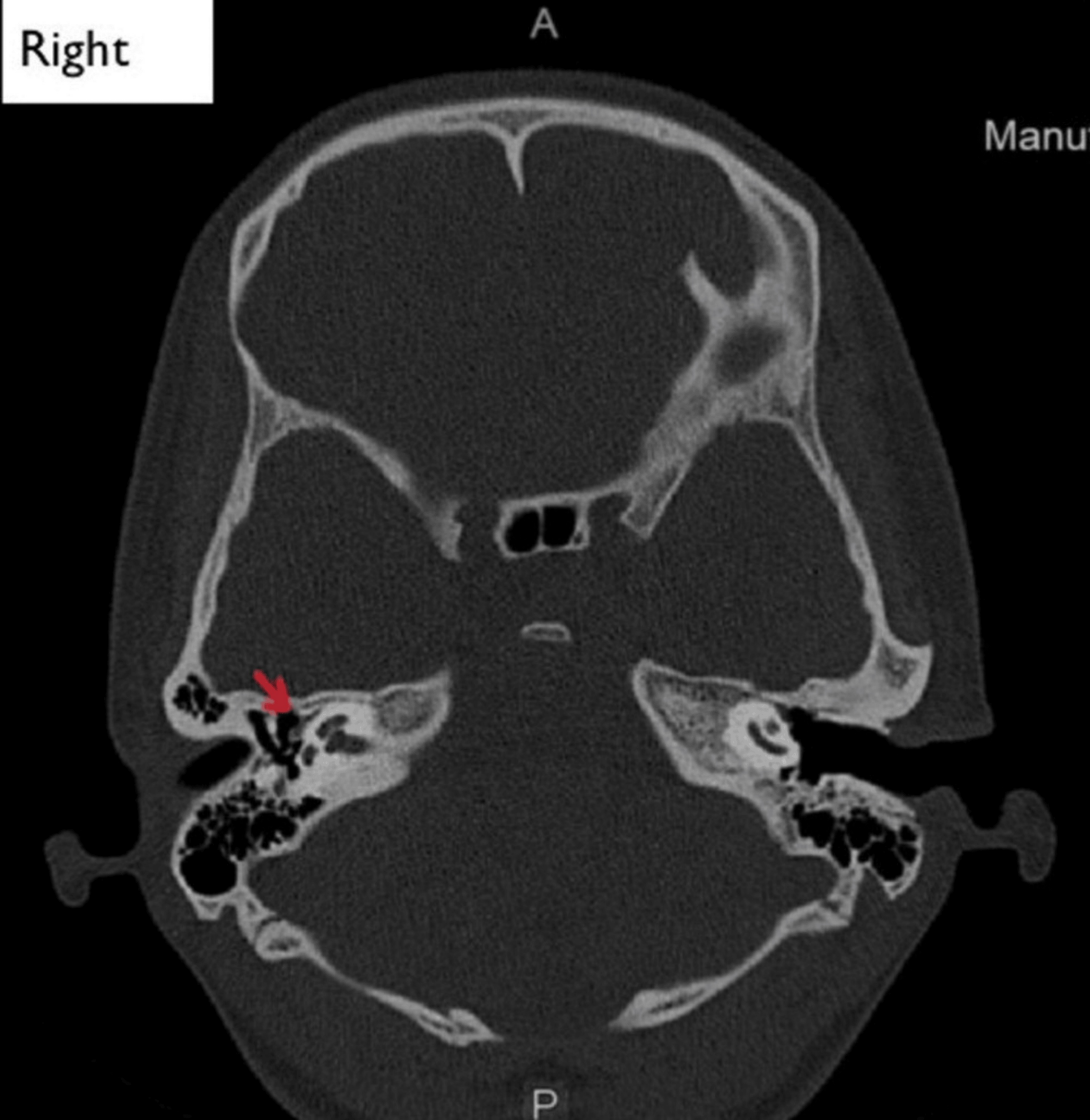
Coronal section of high-resolution computed tomography of the right temporal bone. The red arrow points to the oval window niche and facial nerve.
